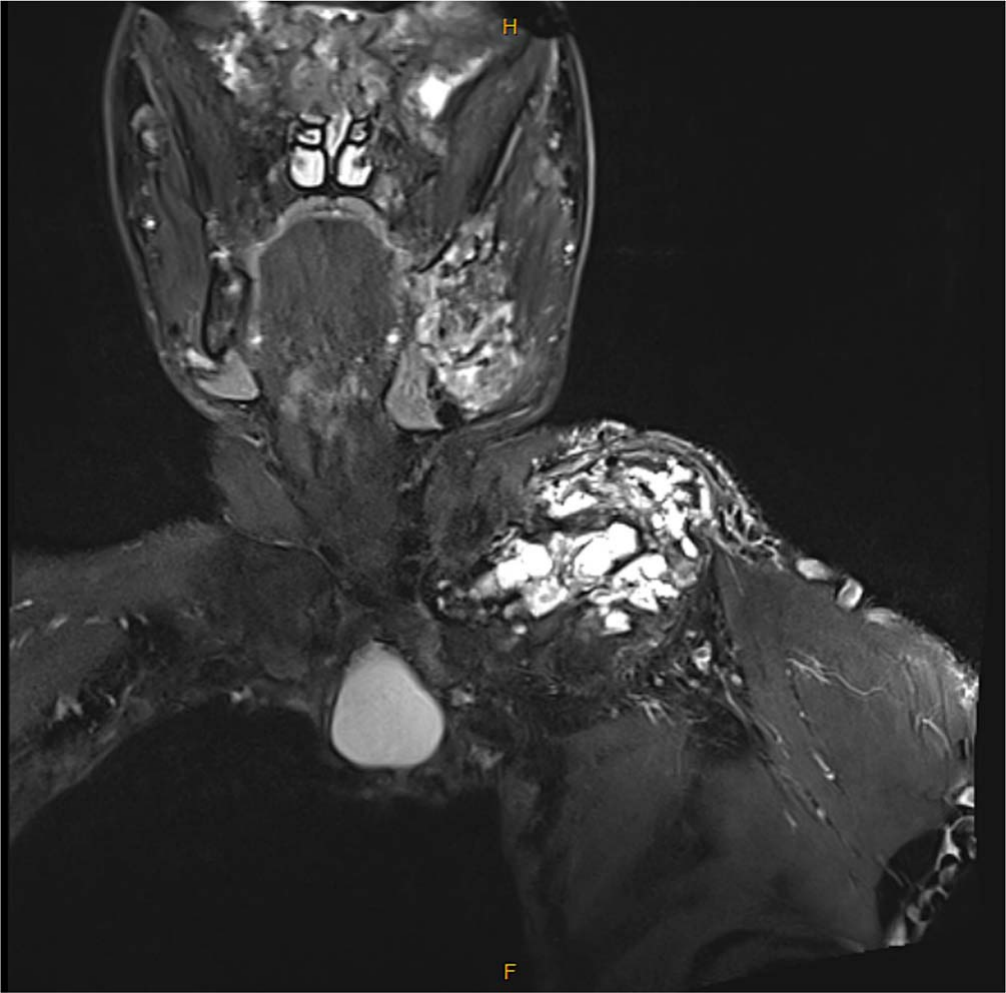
Coronal view of MRI left shoulder and clavicle with large expansile bony lesion on the left clavicle.
